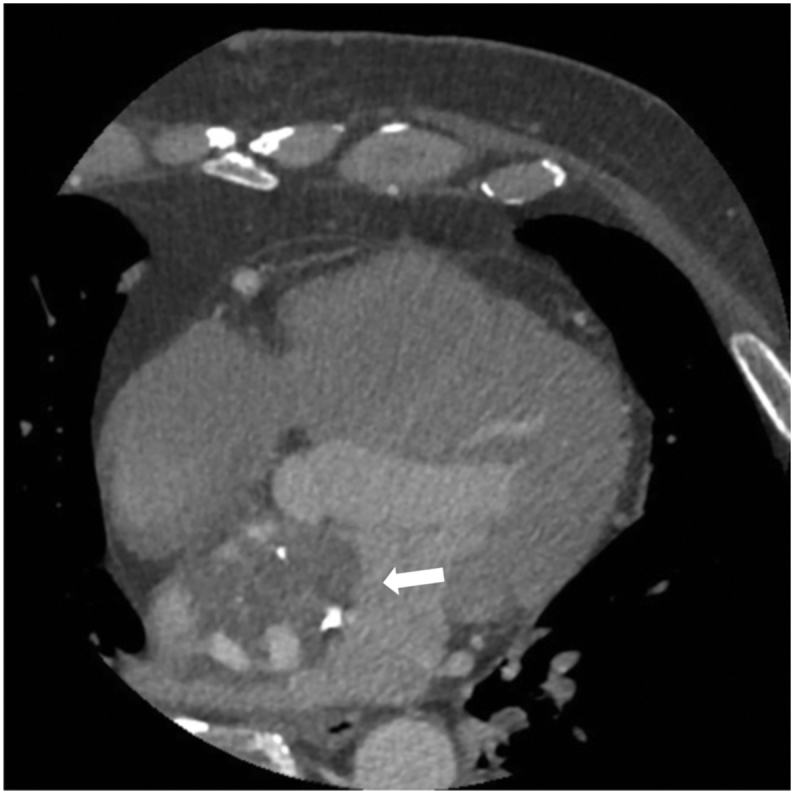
Computed tomography shows a large left atrial thrombus attached to the atrial septum.
